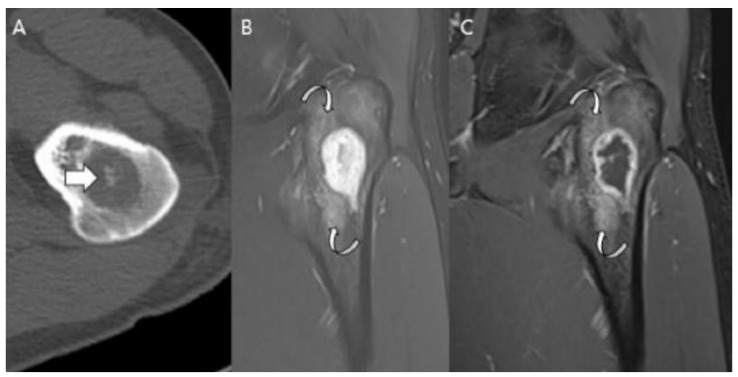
Atypical cartilaginous tumor of the proximal femur in a 22-year-old man. (A) Axial CT scan reveals a low-density intramedullary mass with chondral-type mineralization (arrow) in the proximal femur. (B,C) Coronal T2-weighted fat-suppressed and T1-weighted enhanced images show an intramedullary mass of 3 cm in size with high signal intensity and the peripheral rim and septal enhancement. Note the peritumoral edema with enhancement (curved arrows).
